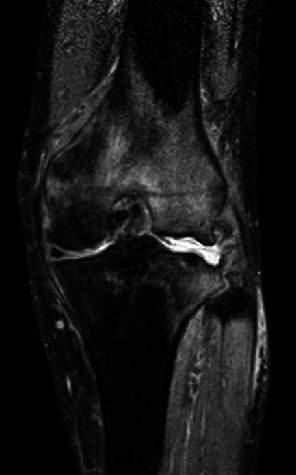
MRI of the left knee. Magnetic resonance image revealing partial depression of the joint surface including shredded ossicles and substantial amounts of synovial fluid. The findings were similar to those of osteomyelitis.
